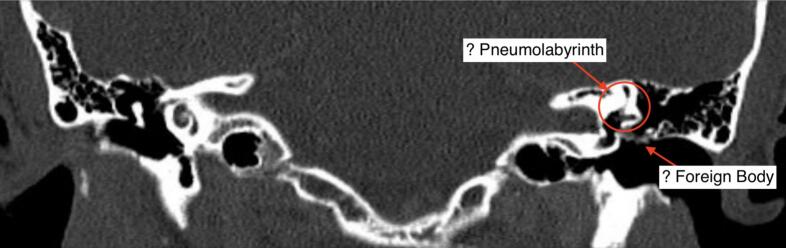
Coronal CT Temporal Bones on bony windows showing airs within the left labyrinthine system.
